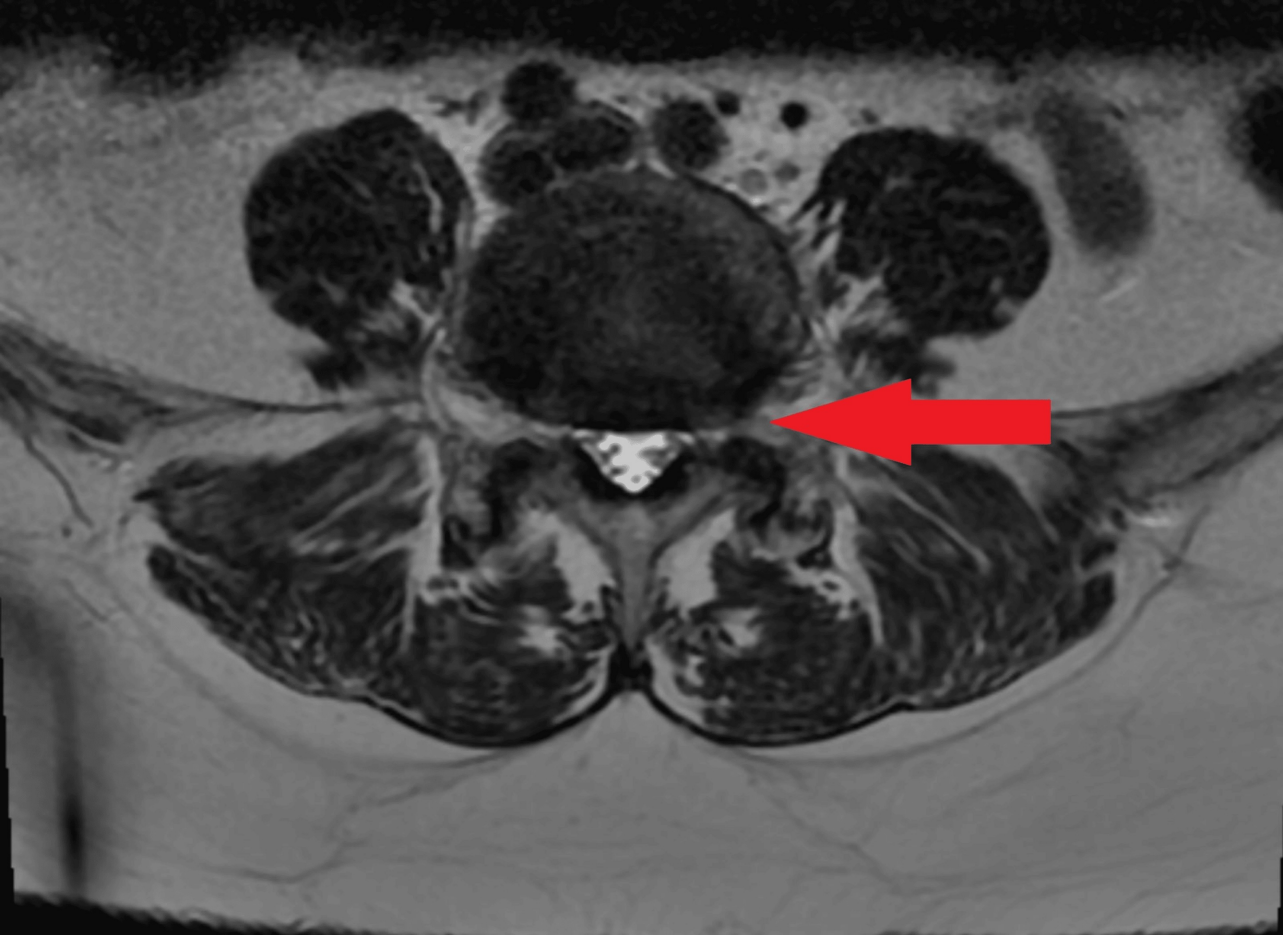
Axial view from lumbar MRI taken 10 months prior to surgery The image shows the L4-L5 disc (arrow) at the site of the spondylolisthesis.
